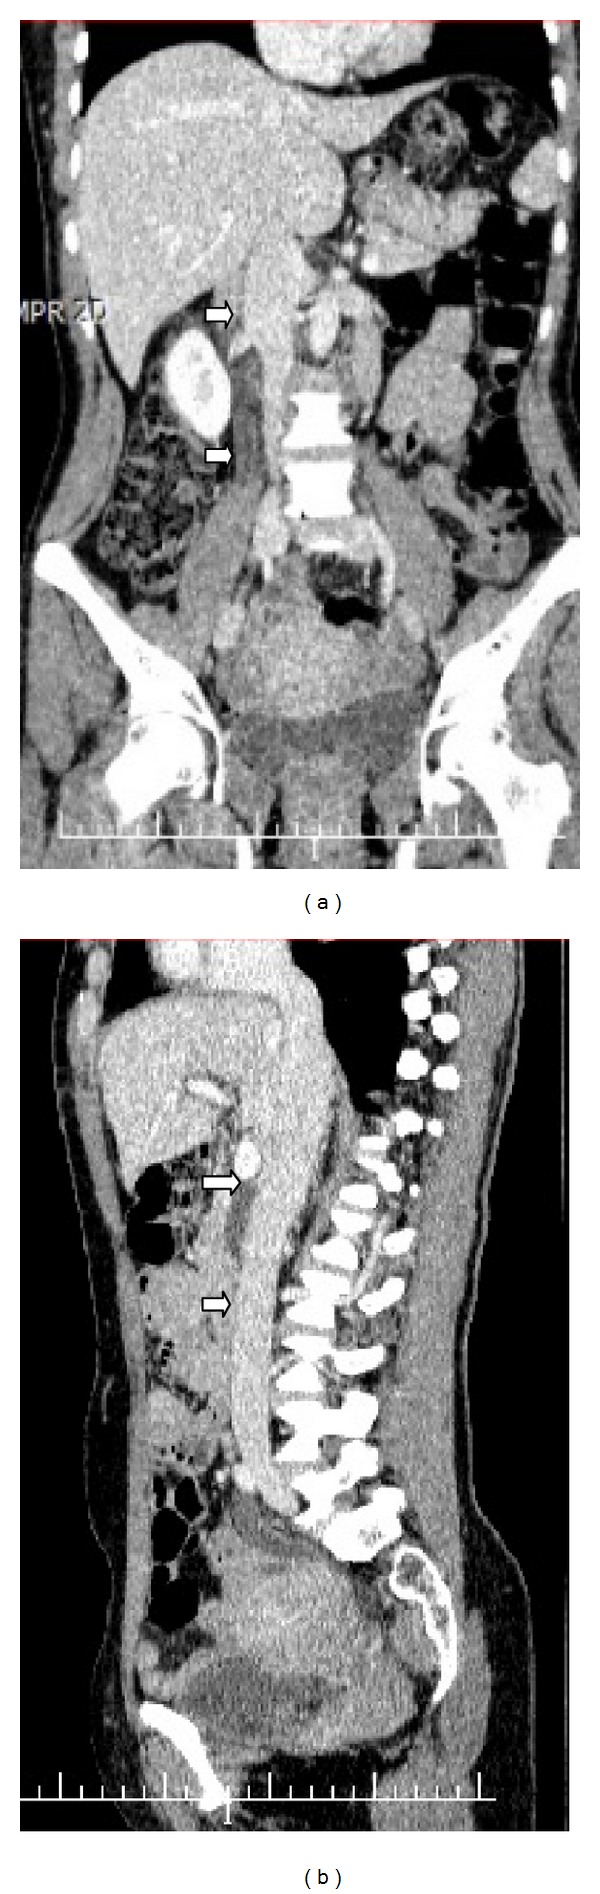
(a) Coronal and (b) sagittal images of CT with contrast enhancement revealed that OV and IVC is patent at the 12th month of OVT with no collateral circulation.
